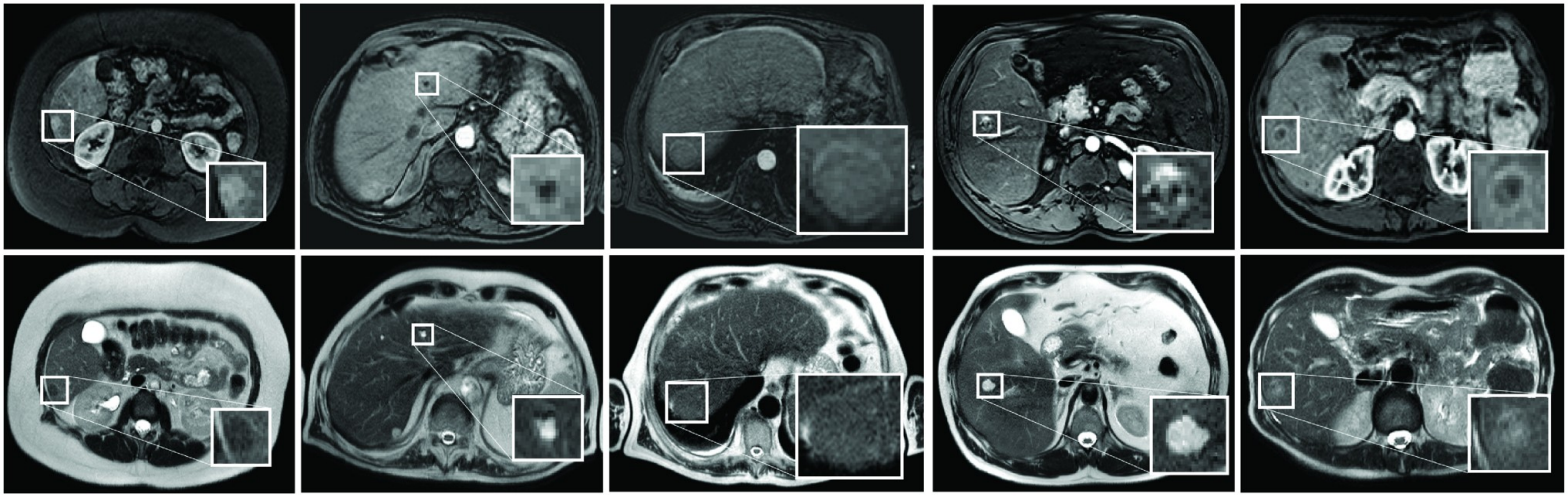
Examples of focal liver lesions. From left to right livers with an adenoma, cyst, HCC, hemangioma, and a metastasis from colorectal carcinoma origin are shown. The top row shows the arterial phases of the DCE-MRI and the bottom row the T2-weighted images. A zoom-in of the lesions is inserted.
